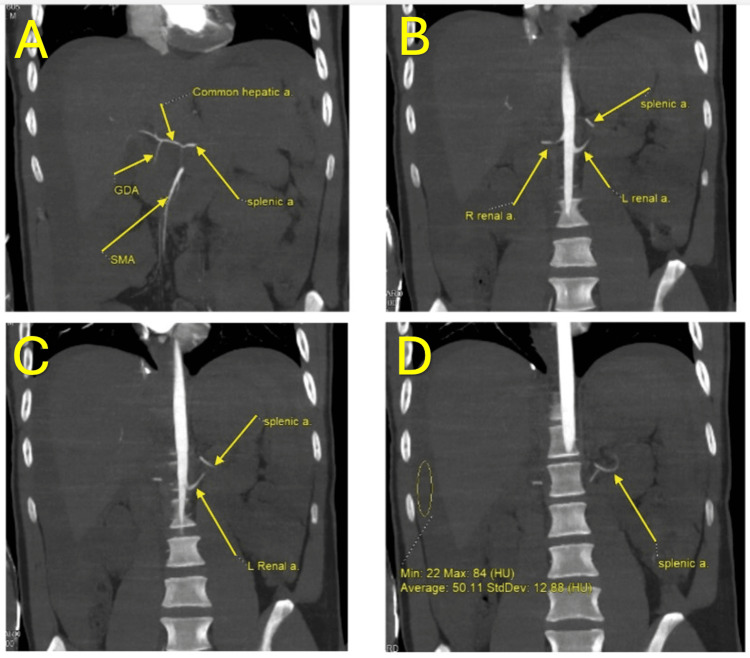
Coronal CTA Demonstrating Hemoperitoneum Without an Identifiable Vascular Source Coronal computed tomography angiography (CTA) images demonstrating intraperitoneal fluid concerning for hemoperitoneum without an identifiable vascular source of hemorrhage. (A) Coronal image of the celiac axis with labeled common hepatic artery, gastroduodenal artery (GDA), superior mesenteric artery (SMA), and splenic artery. (B–D) Sequential coronal images demonstrating the course of the splenic artery (arrows) without evidence of aneurysmal dilation or active contrast extravasation. In panel D, the circled region of intraperitoneal fluid demonstrates an average attenuation of approximately 50 Hounsfield units, supporting hemorrhagic ascites/hemoperitoneum.
